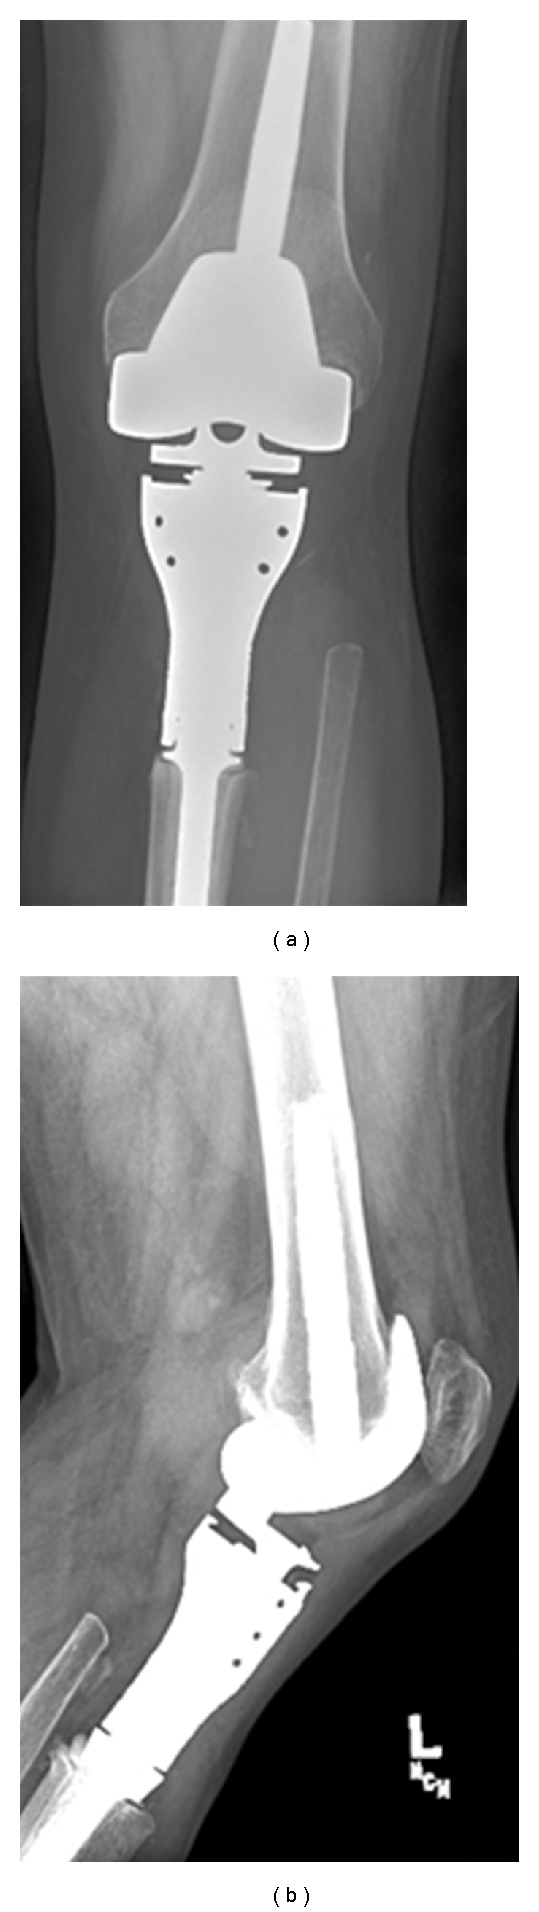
Postoperative anterior posterior, (a) and lateral (b) radiographs of the left knee demonstrate the endoprosthetic reconstruction of the proximal tibia and knee joint.
